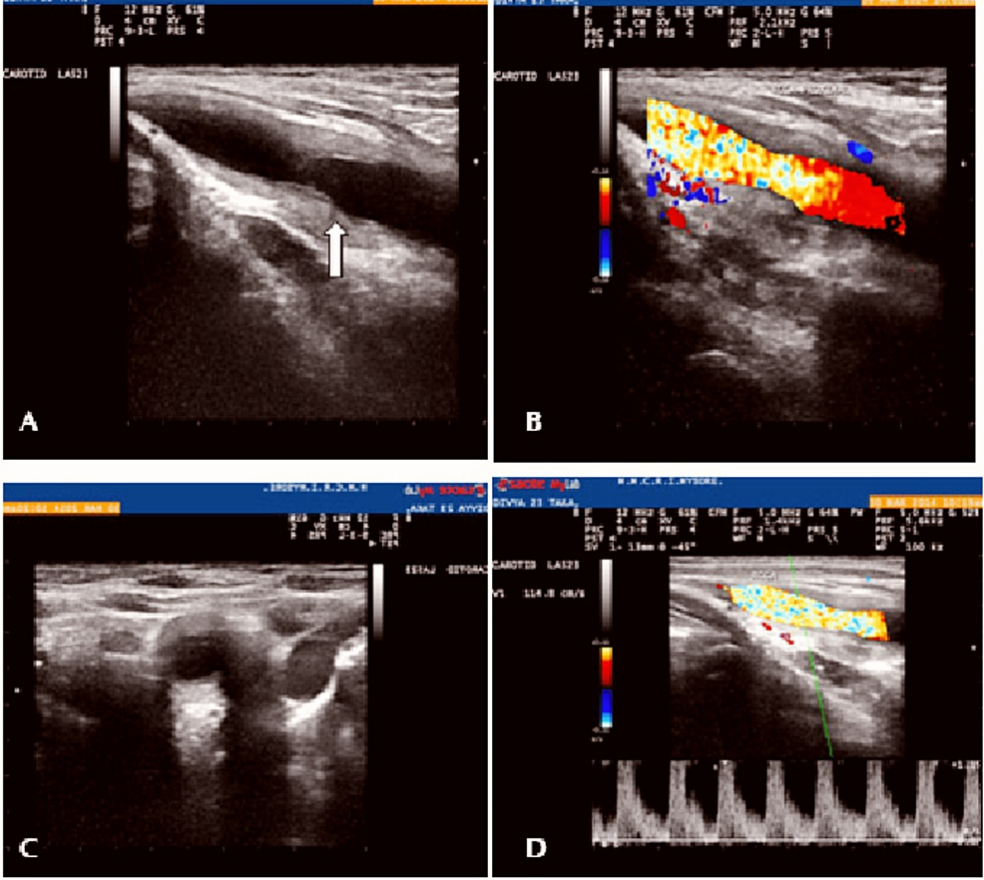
Right common carotid artery Doppler Long segment (shown by white arrow) diffuse homogenous wall thickening, spectral broadening of waveform in common carotid artery (CCA)
